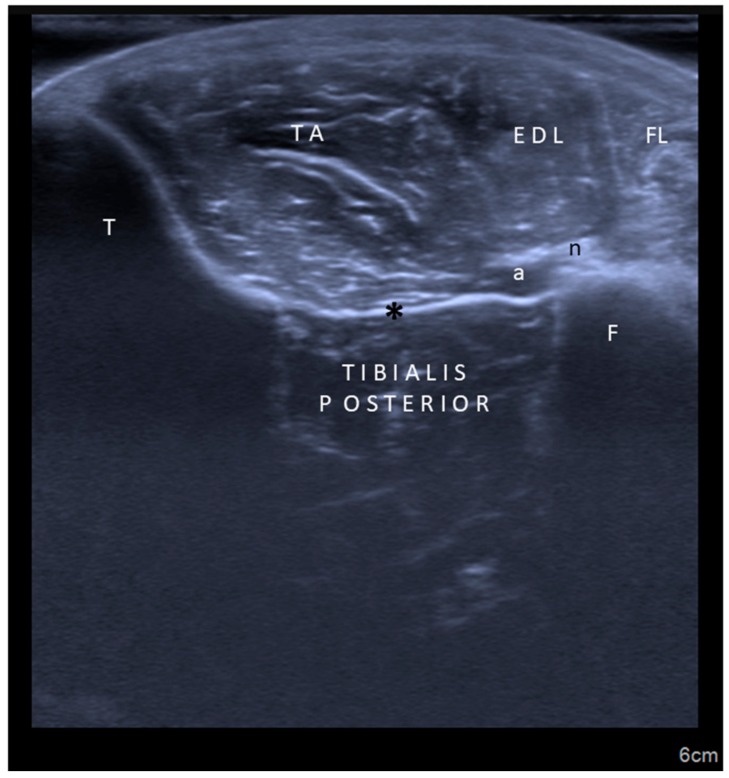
Ultrasound imaging (axial view) for tibialis posterior muscle. TA: Tibialis anterior; T: Tibia; F: Fibula; EDL: Extensor digitorum longus; FL: Fibularis longus; a: Artery; n: Nerve; *: Interosseous membrane.
